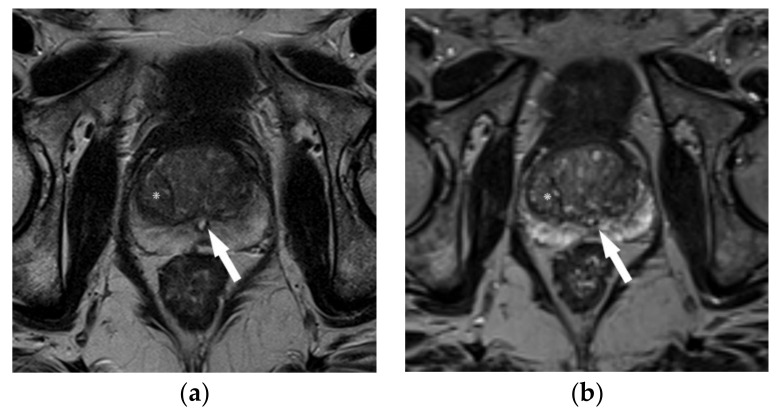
58-year old patient with elevated PSA undergoing MRI before template biopsy. Anatomic landmarks such as the verumontanum (arrow) and BPH-nodule (*) are more clearly depicted on 2D TSE acquisition (a) than on isotropic 3D sequences (b).
